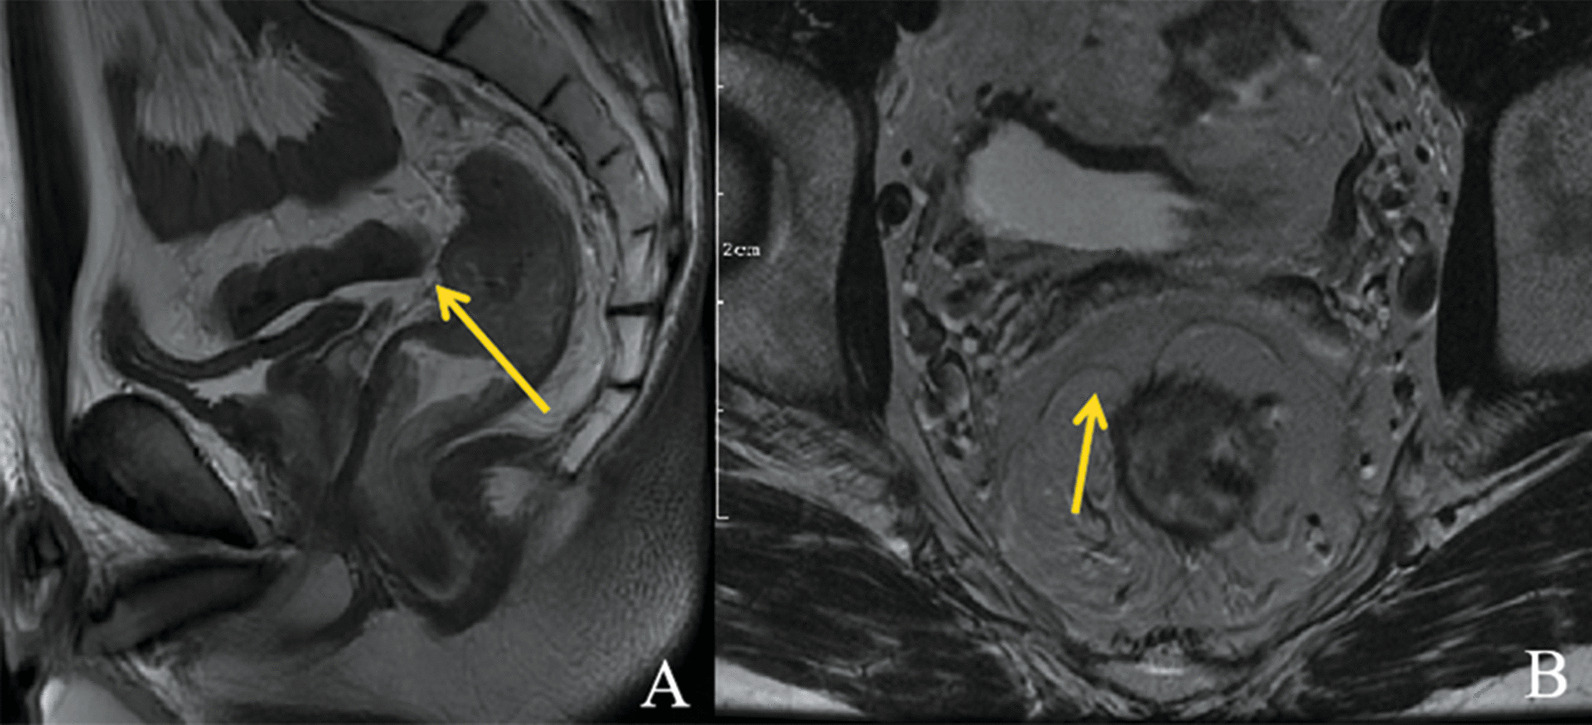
Identification of the APR on MR imaging. a Sagittal plane: the APR was a thin hypointense linear structure noted along with the superior bladder, which extended inferiorly and posteriorly to the tip of the seminal vesicles, after which the posterior extension attached to the anterior rectal wall. b Axial plane: the APR attaches to the anterior rectal wall in a V-shaped hypointense configuration. APR: anterior peritoneal reflection
